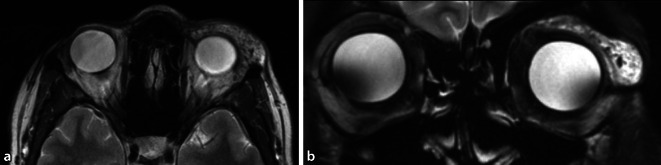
Es erfolgte eine Doppler- und farbkodierte Duplexsonographie zur Beurteilung der Durchblutungssituation („high-flow“ vs. „low-flow“). Diese ergaben eine deutliche Hyperperfusion mit schneller Strömungsgeschwindigkeit („high flow“) in den zuführenden Gefäßen.
